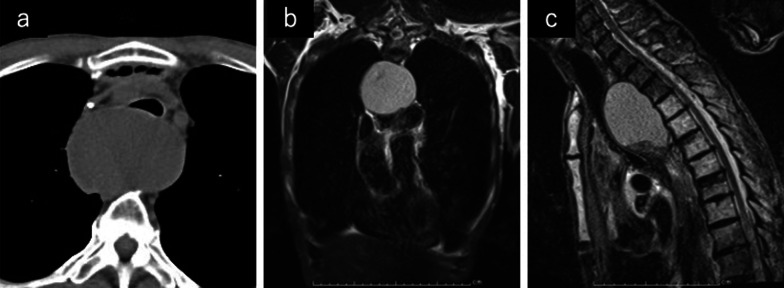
Mucocele of the esophagus. a CT scan showing tracheal compression due to the mucocele. b MRI showing the presence of fluid collection and dilatation of the remnant esophagus. c MRI showing tracheal compression due to the mucocele on the sagittal view
